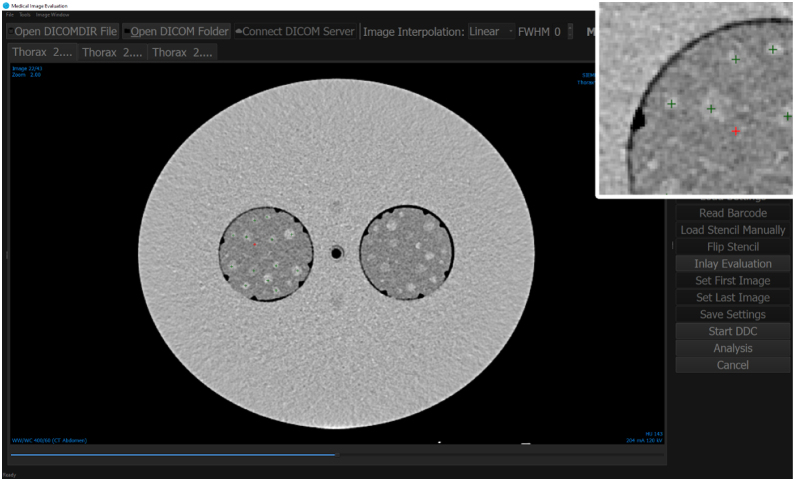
Analysis of the DDC modules from an CT scan with both modules from an Siemens Somatom Definition AS with a chest scan protocol, 120 kV tube voltage and 294 mA tube current. The module with contrast agent (no. 1) is on the left side while the native DDC module (no. 2) is on the right side. An abdomen window (ww:400 and wc:60) is used. The figure shows our software “RadiVates”. The overlay at the top right was magnified by 300%. The green crosses are contrast objects which were seen by the observer while the red cross designates a false click. The software runs in dark mode during the DDC evaluation.
